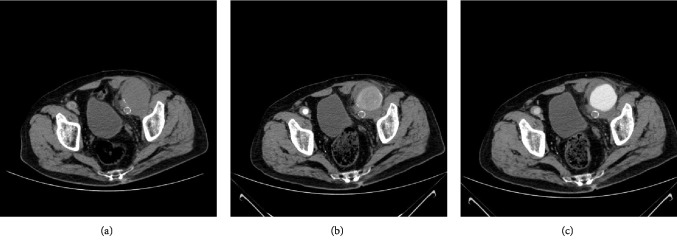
Same axial slice using a 64-detector row CT scanner (a) before and (b) & (c) after contrast media injection (arterial and portal phase respectively), revealing a ruptured left femoral pseudoaneurysm at the distal end of the left limb of the aortobifemoral graft.
